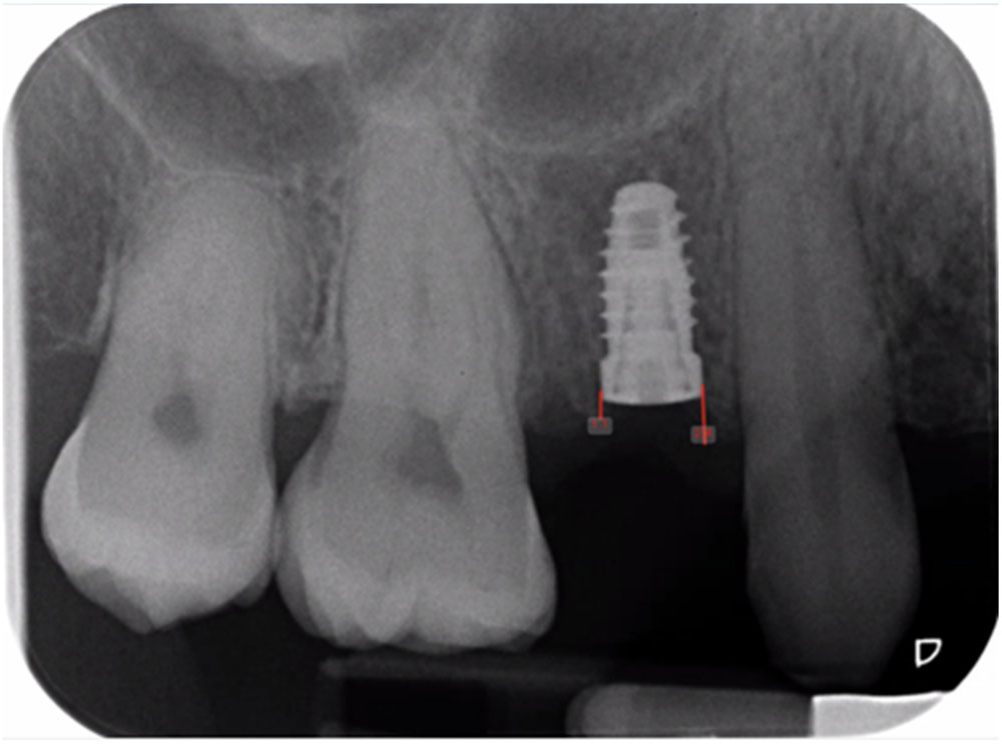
Radiographic measurement of marginal bone levels. Mesial and distal measurements were taken at each implant from the platform to the first bone contact.
